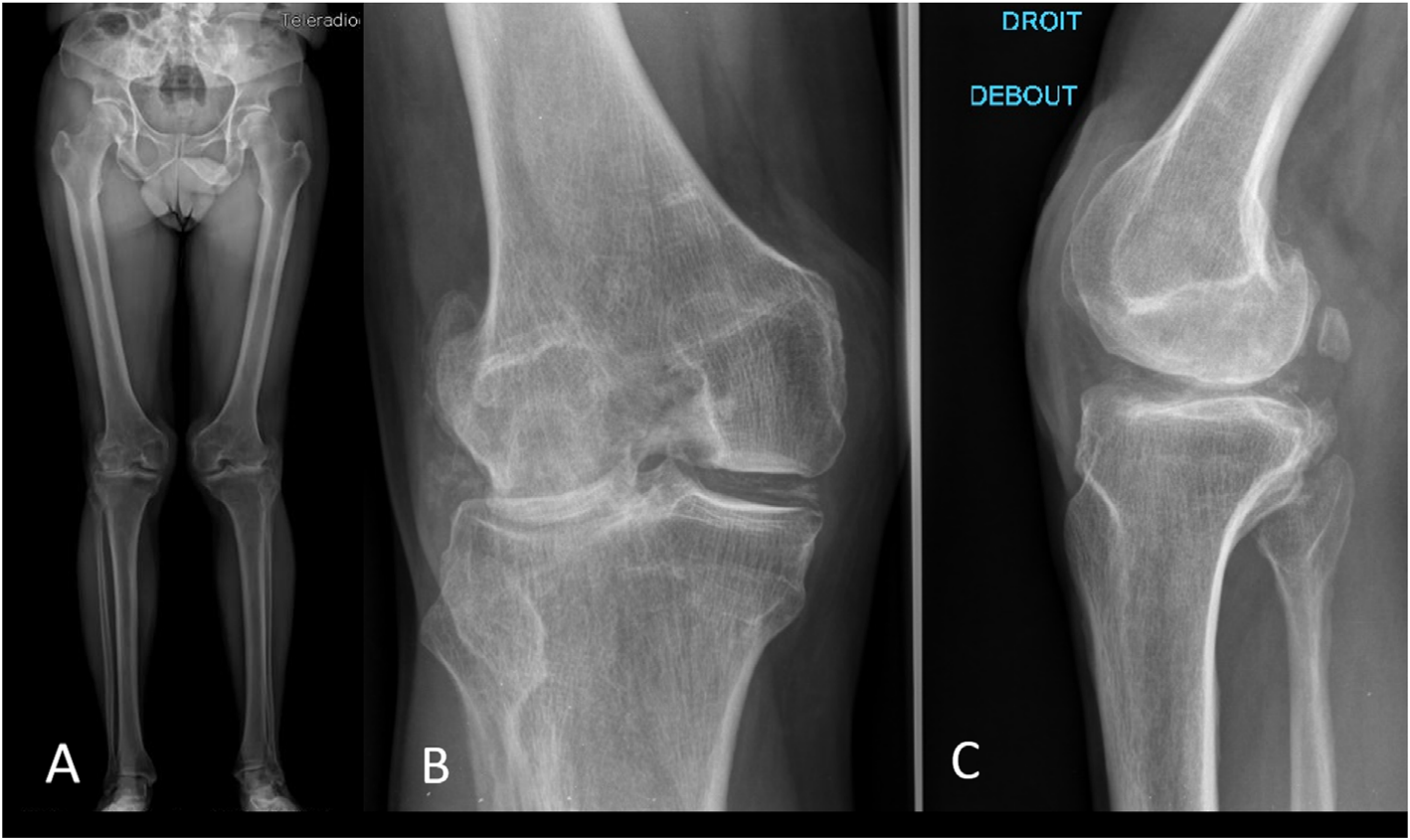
Pre-operative plain radiographs: (A) standing both lower limbs, (B) anteroposterior (AP), (C) lateral view, right knee.
